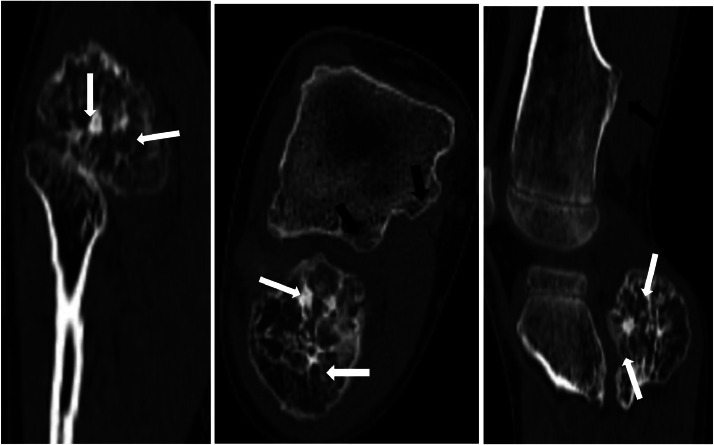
CT coronal, axial and sagittal slices show a heterogeneous fibular bone outgrowth with irregular contours and calcifications (whites arrows), and other homogeneous tibial bone outgrowths with the same structure as the adjacent bone (blacks arrows).
